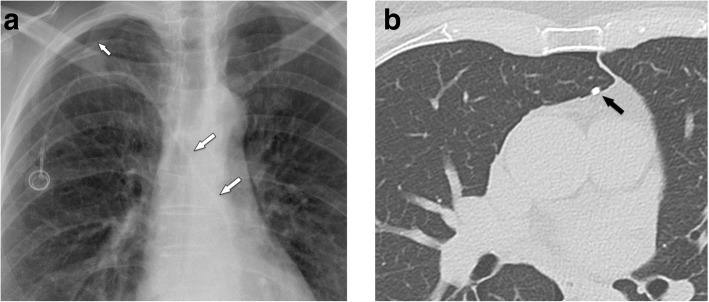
Malpositioning in the pleural space. a Postoperative chest radiograph shows an abnormal course of the venous catheter, which crosses the midline. A small apical pneumothorax is noted (arrow). b Subsequently, a MDCT was performed, which shows the venous line in the pleural space (arrow) and a pneumothorax
